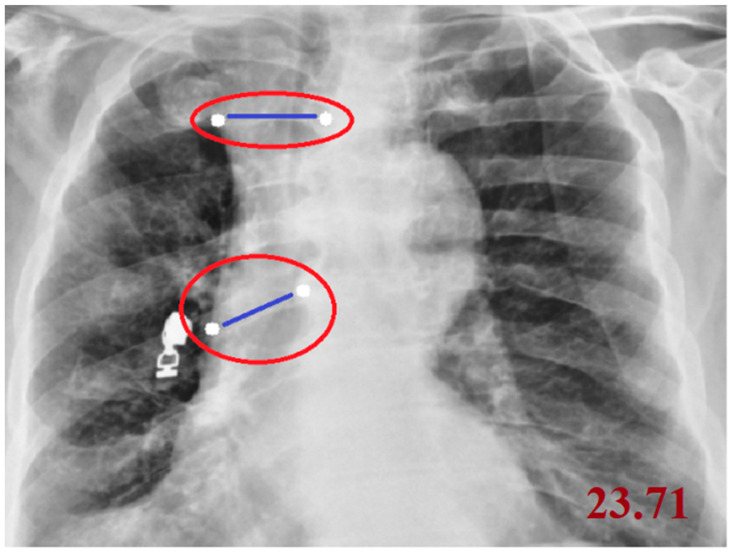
Measurement of the Cobb Angle on a Posteroanterior Chest Radiograph.
